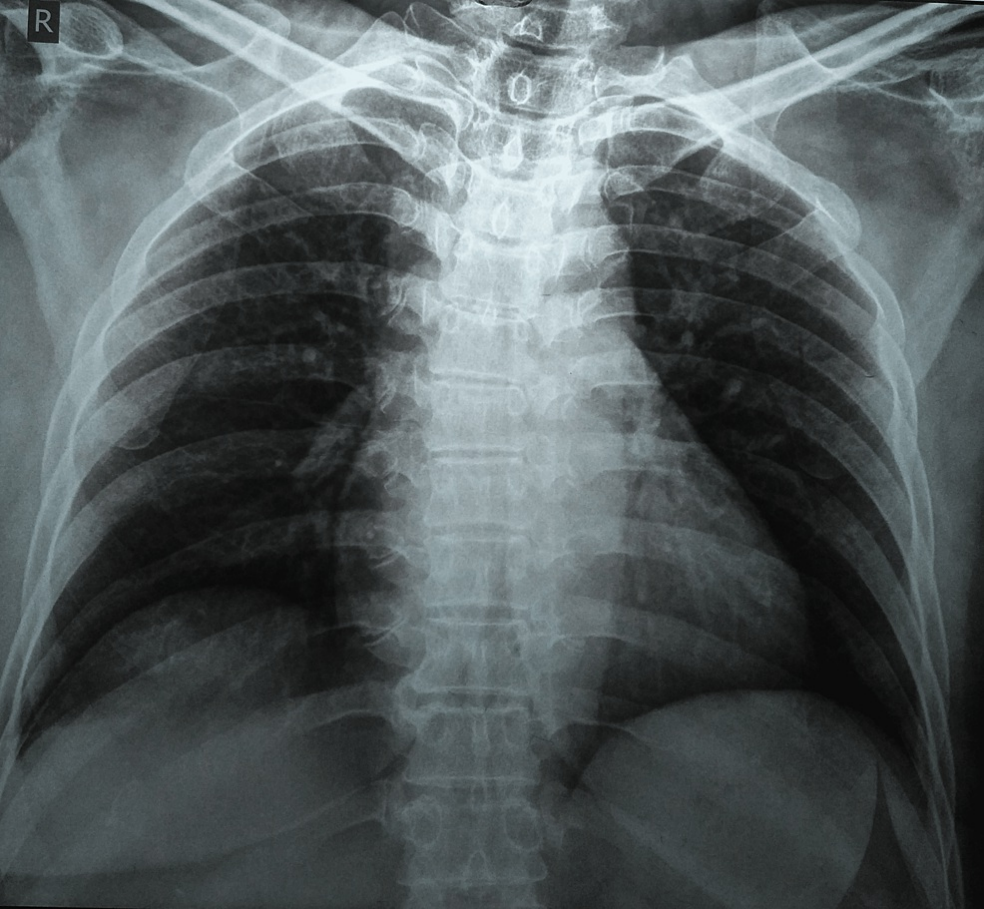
A chest radiograph (A-P view) within normal limits A-P: antero-posterior
